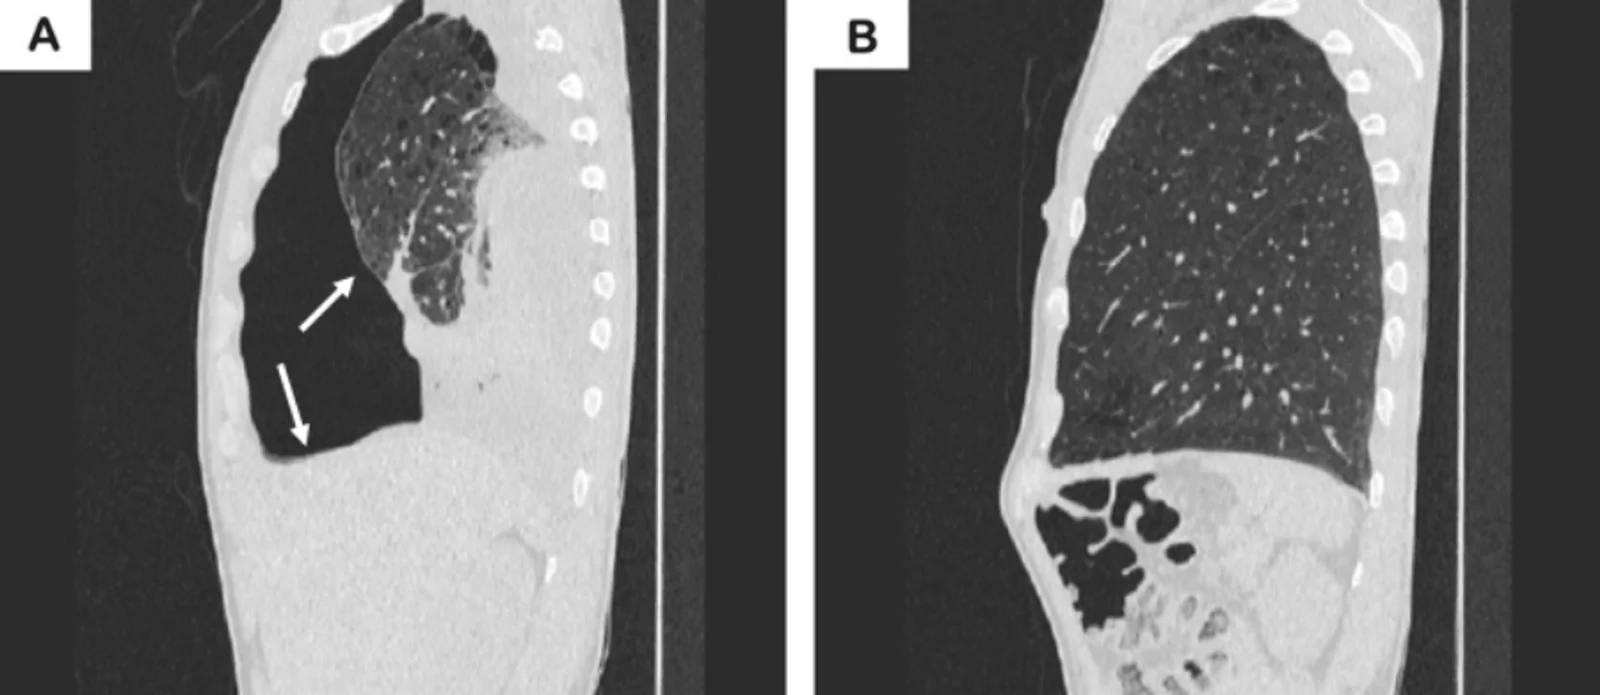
Sagittal chest computed tomography comparison, taken on day one of admission. (A) Sagittal slice of the affected hemithorax demonstrates a massive compressive pneumothorax (upper white arrow) displacing the collapsed right lung tissue, along with a significant dependent pleural effusion (lower white arrow). (B) Contralateral sagittal slice demonstrates the normal left side, fully expanded lung parenchyma, and a clear pleural space for anatomical comparison.
